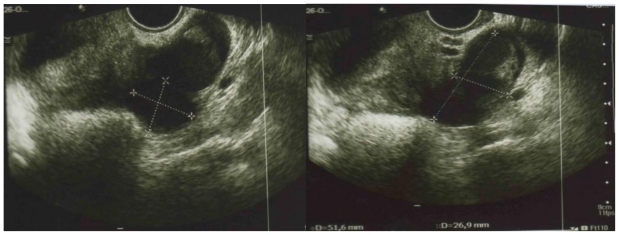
Transvaginal pelvic ultrasound shows cystic structures with low level internal echoes and echogenic wall foci, thickened walls and septations.
